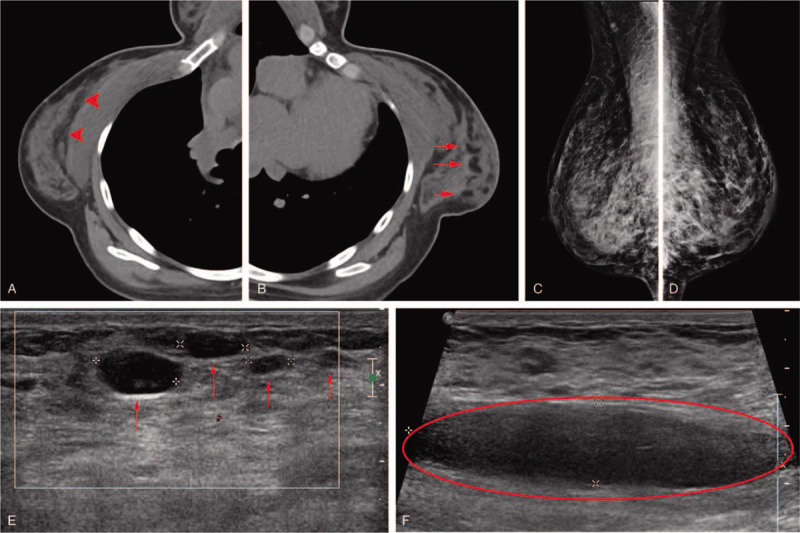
(A, B) Computed tomography scan. Multiple cystic lesions and fluid-filled areas were observed in both breasts (arrow). (C, D) Mammography. (E) Ultrasonography. Multiple hypoechoic lesions suggestive of cystic masses were observed in the breast parenchyma (arrow). (F) Ultrasonography. Fluid collection was observed in the retromammary space (circle).
